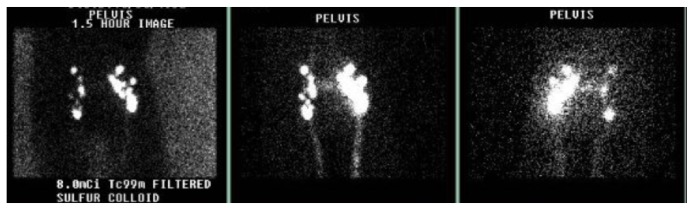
Lymphocytic scintigraphy results of the pelvic area showing no activity beyond the pelvis.
